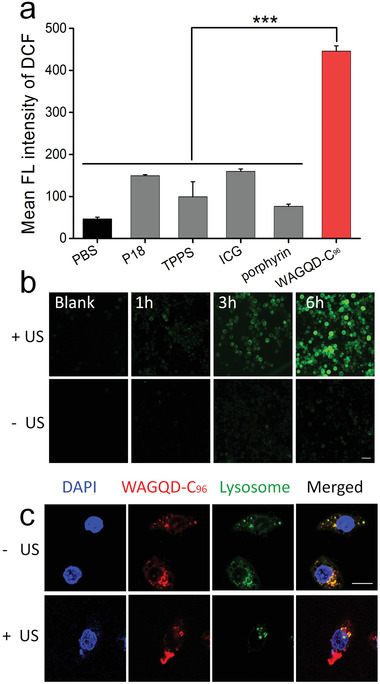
In vitro sonosensitization of WAGQD‐C_96_. a) ROS generated levels from WAGQD‐C_96_ and other sonosensitizer [purpurin 18 (P18), meso‐tetrakis (4‐sulfonatophenyl) porphyrin (TPPS), ICG, and porphyrin] at 20 µm concentration under ultrasound at 0.56 W cm^−2^ for 5 min. PBS: phosphate buffer saline. All data present as means ± SD (n = 3). P‐values are calculated using one‐way ANOVA with GraphPad Prism Software, p* < 0.001. b) Cellular ROS generation of WAGQD‐C_96_ with/without ultrasound treatment at 0.56 W cm^−2^ for 5 min. Scale bar: 100 µm. c) Lysosome escape in the presence of WAGQD‐C_96_ with/without ultrasound treatment at 0.56 W cm^−2^ for 5 min. Scale bar: 20 µm.
